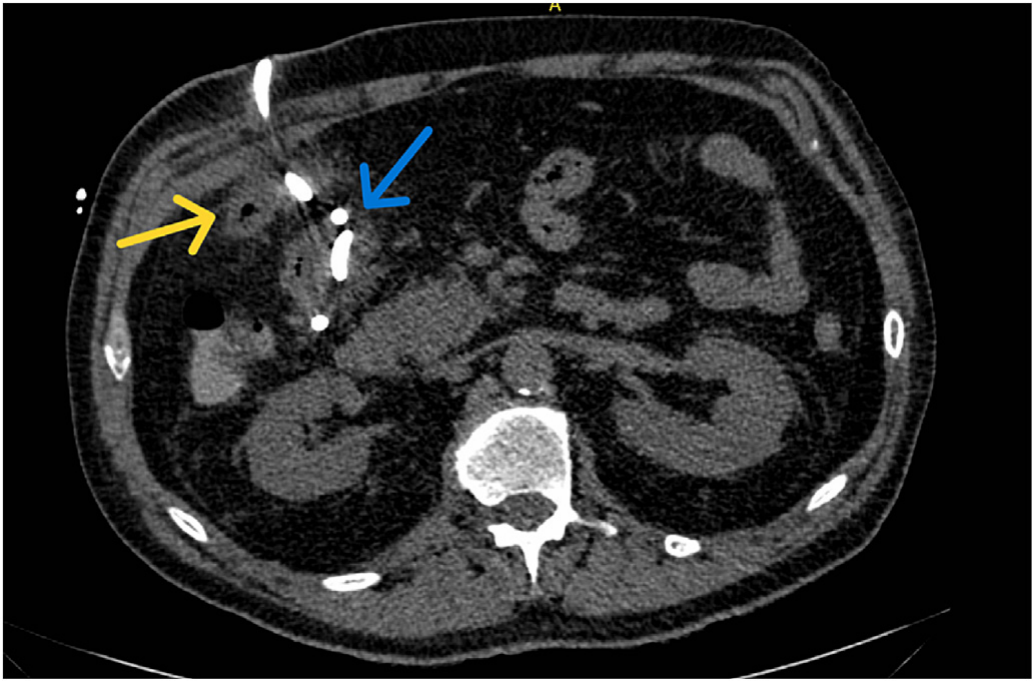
Axial non-contrast CT abdomen after treatment showing post-surgical changes of cholecystectomy and right upper quadrant abscess pigtail drain placement (blue arrow) with interval decreased size of the previously seen right upper quadrant abscess which closely abuts the hepatic flexure of the colon (yellow arrow).
